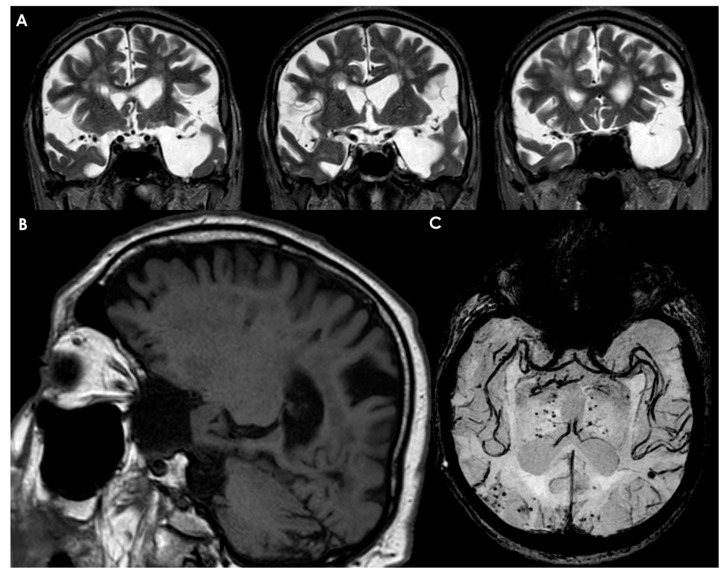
Brain MRI (1.5 T) of a 85 years old patient with a diffuse cerebral atrophy and a left temporo-polar arachnoid cyst (coronal T2W in panel (A), sagittal T1W in panel (B) and Susceptibility Weighted Imaging reconstructed according with Minimum Intensity Projection and MPR protocols in panel (C)). Middle cerebral artery on both sides is coursing within an enlarged sylvian cistern and close to a CSF-filled temporo-polas arachnoid cyst on the left side.
